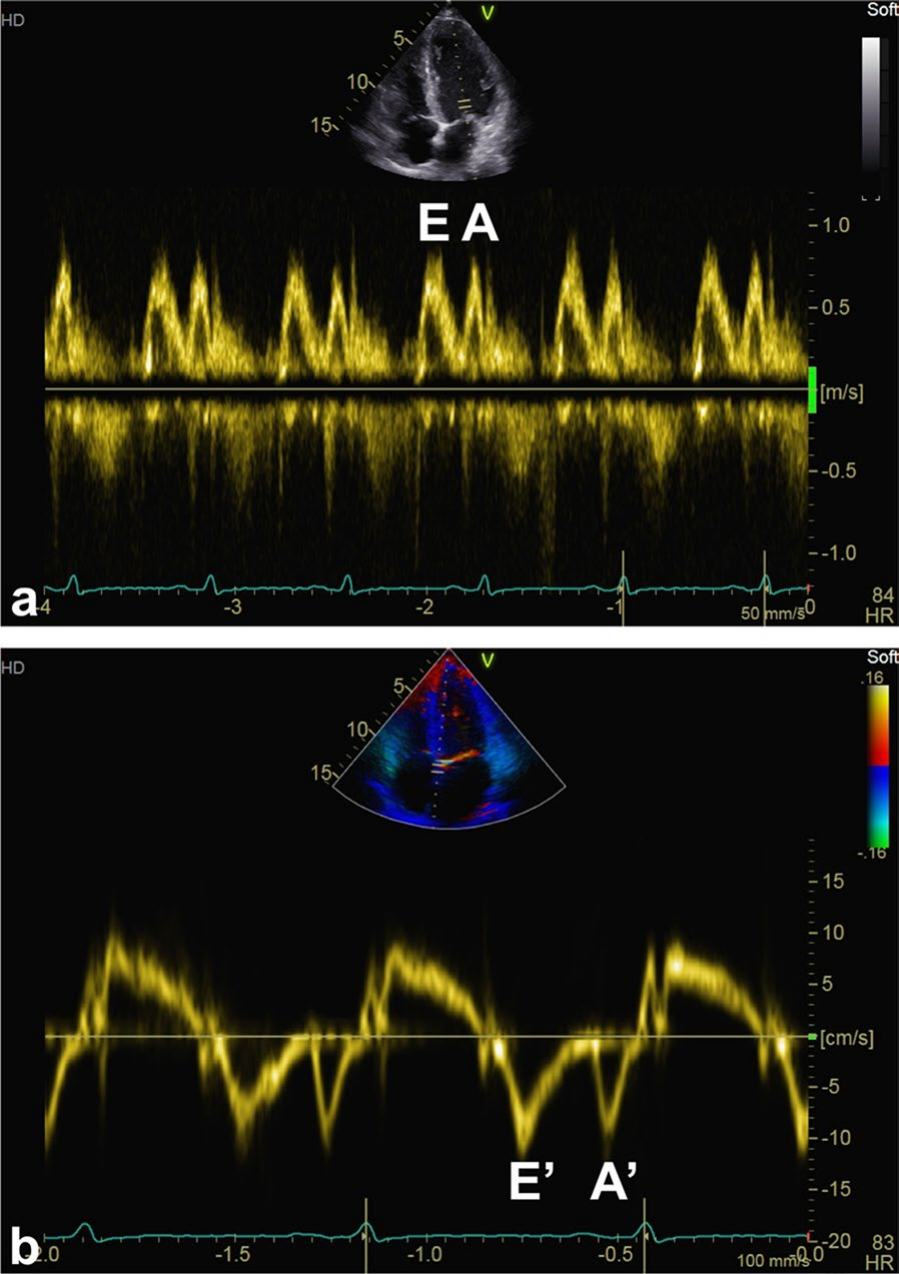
Pulse wave Doppler and tissue Doppler. a Trans-mitralic Doppler; an early wave (E wave) is displayed on the monitor that represents the first phase of the diastole as a passive phenomenon, limited only by the stiffness of the left ventricle or by interventricular interdependence—right ventricular push over the left ventricle via the septum. The E wave in patient with sinus rhythm is followed by atrial contraction (A wave). b Septal tissue Doppler; tissue Doppler directly measures myocardial velocities by means of the Eʹ wave in a load-independent way. The combination of the trans-mitral Doppler E wave, the tissue Doppler Eʹ wave, and the *E*/*E*ʹ ratio at the end of an SBT also seems to be capable of predicting weaning failure from diastolic dysfunction
